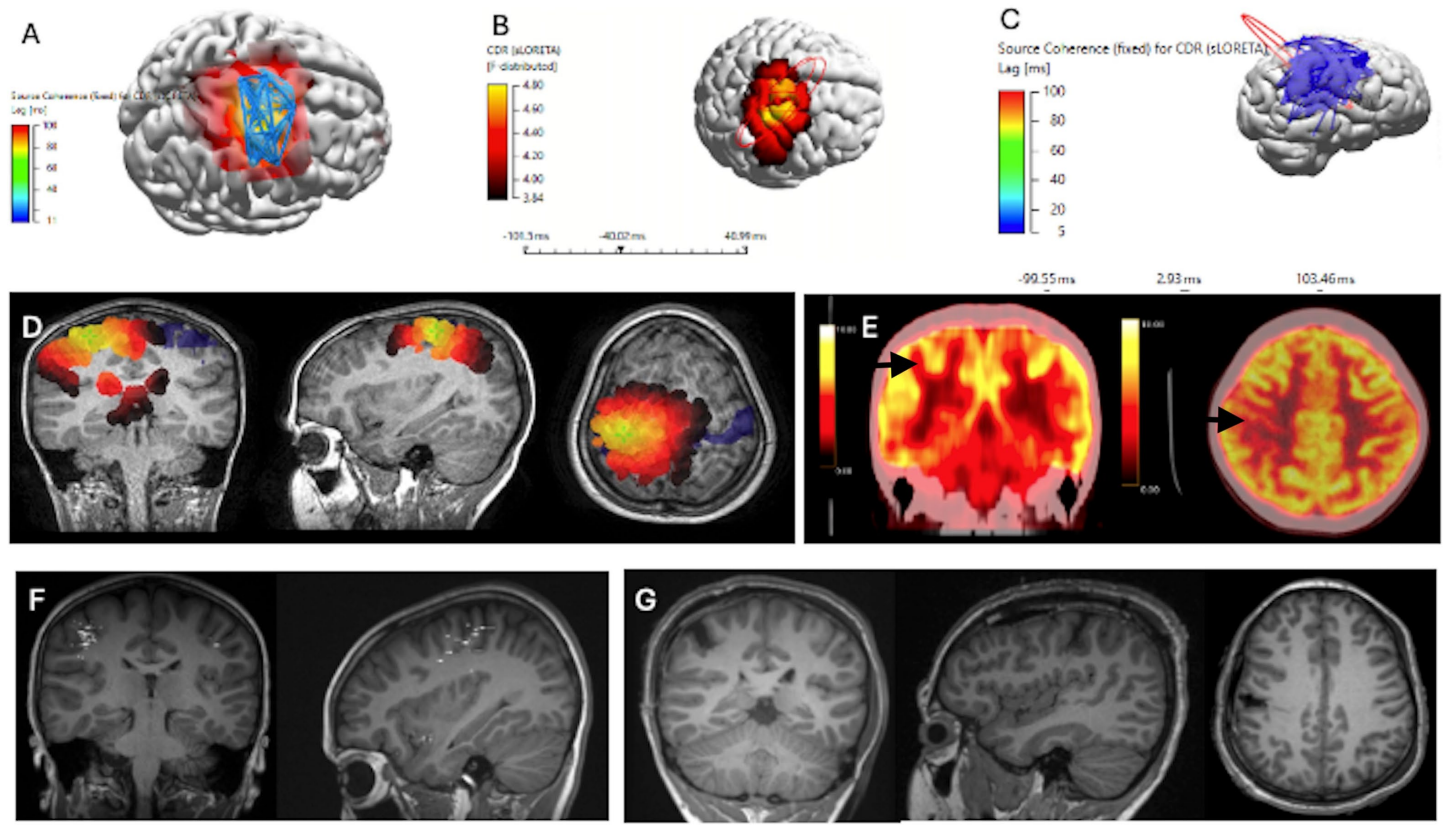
Concordant Localization of Ictal ESI Source Coherence, Interictal HFO ESI and Interictal HFO Source Coherence to Resection Margins, for ILAE Class 1 Patient, alongside MEG and FDG PET scans (Case 2). Patient underwent a lesionectomy of a right post-central gyrus lesion. (A) Ictal ESI Source Coherence Map localizing to the right pre-central and post-central gyrus. (B) Interictal HFO ESI localizing to the right post-central gyrus. (C) Interictal HFO Source Coherence map identifying a network localizing to the right parietal, central and temporal regions. (D) sLORETA source localization through ICA, clipped at 75%. (E) FDG-PET scans identifying a region of hypometabolism within the right post-central gyrus. (F) Interictal MEG Dipole Cluster localizing to the right frontal, temporal, and parietal regions. (G) T1 MRI Images of Post-Operative Resection Margins with Coronal, Sagittal and Axial views.
